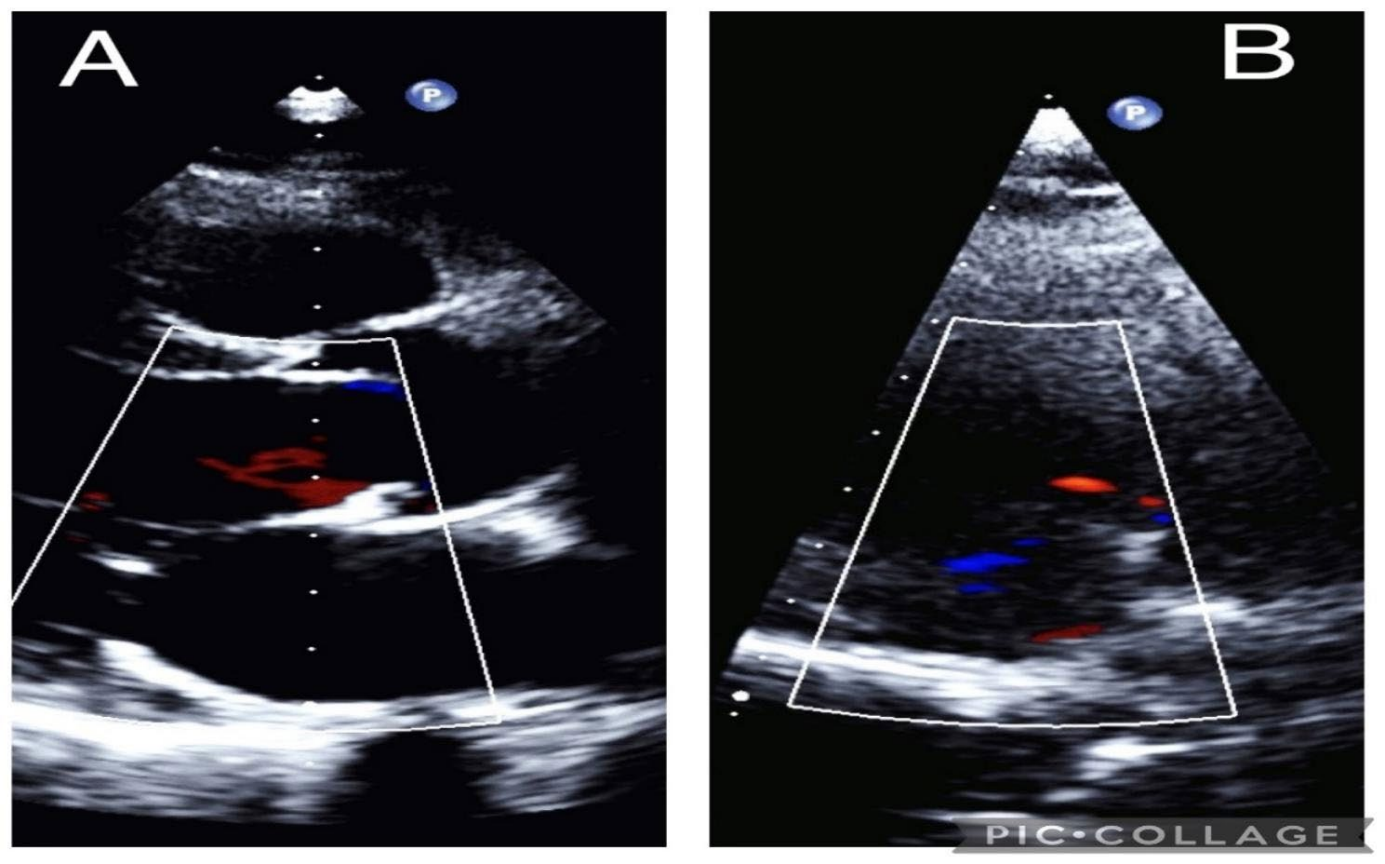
The echocardiogram conducted 6 months after the surgery revealed no indications of new tumor growth (A: Case 1, B: Case 2)
